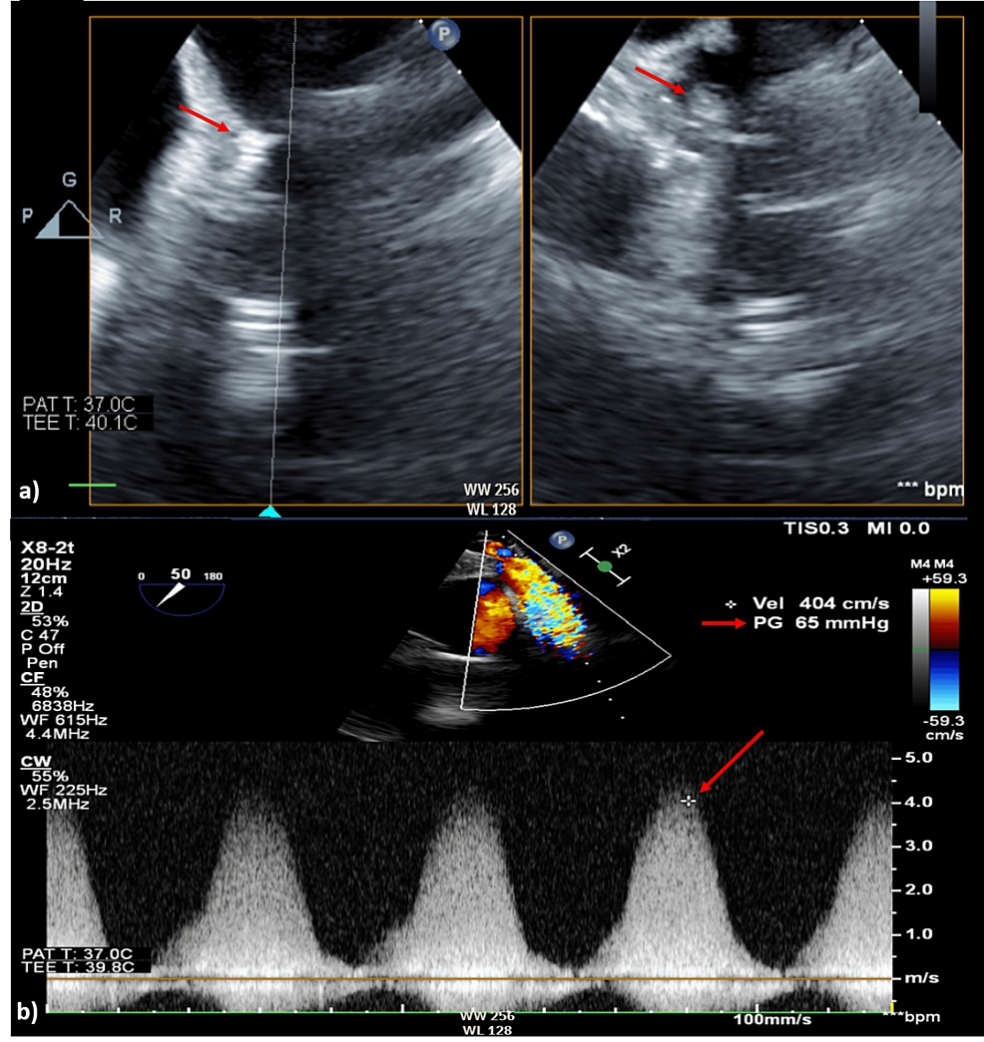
Transthoracic echocardiogram conducted on January 5, 2023. (a) A mobile mass at the mouth of the prosthetic pulmonary valve (PPV1) from the right ventricular perspective, indicative of vegetation (arrows). (b) Substantial obstruction (peak gradient of 65 mmHg). The flow navigates around the mass, resulting in severe pulmonary regurgitation (arrows).
